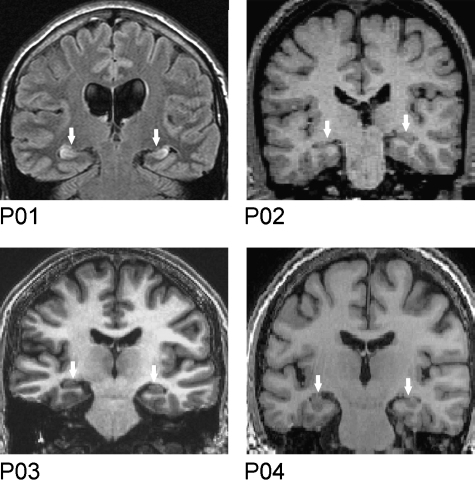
Structural MRI scans: coronal sections through the MRI brain scans of each patient, where the damaged hippocampi are indicated by white arrows. Note P01's scan is a FLAIR image, while the other scans are T1 images.
